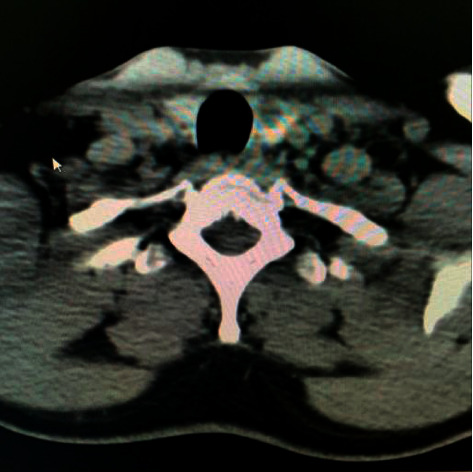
Axial cross section of a soft-tissue window CT image of the left thyroid nodule.
